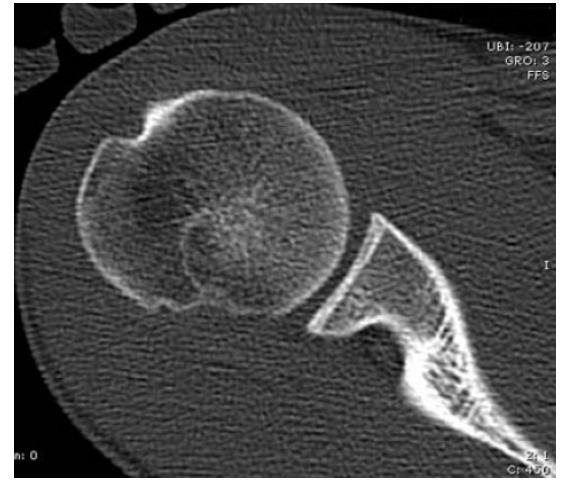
Computed tomography of the shoulder of a patient with shoulder instability showing a small Hill-Sachs lesion.
